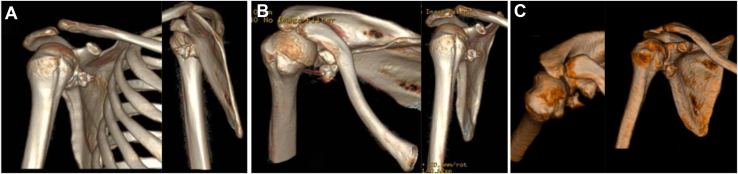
Post-operative 3D-CT scan. (A) Complete healing. (B) Partial healing. (C) No healing. *3D*, three-dimensional; *CT*, computed tomography.
